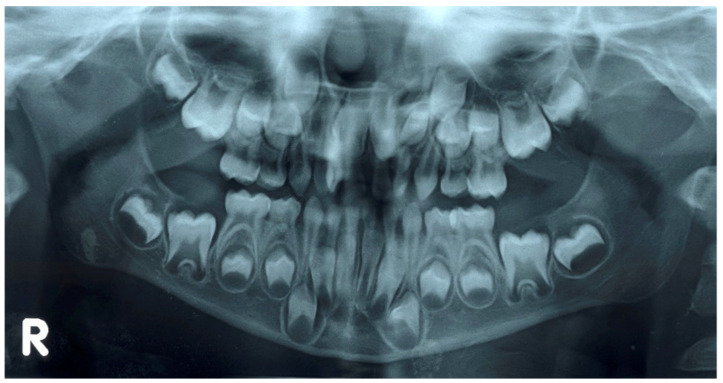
Example of Orthopantomography of patient with supernumerary tooth.
